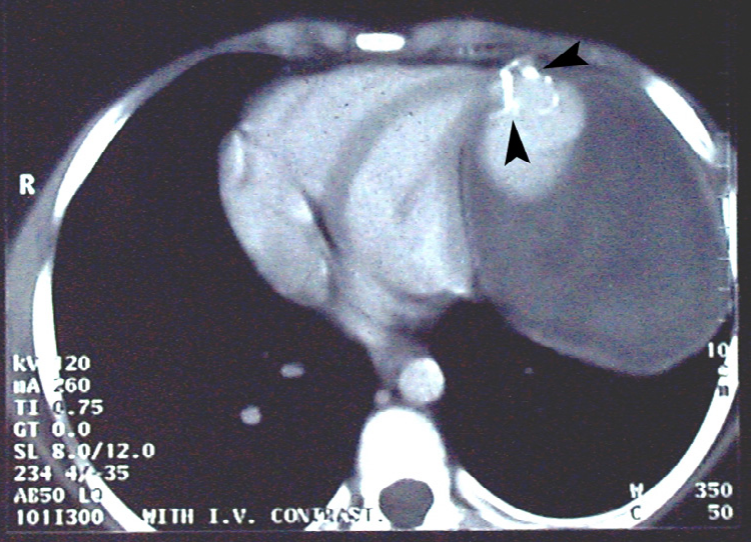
Chest computed tomography scan with contrast at the level of T7 showing the large pseudoaneurysm. The lesion was a well-defined partially calcified mass with tubular density adjacent to the heart. Arrowheads indicate the calcifications. It was filled with contrast medium concurrently with the heart. This lesion, which was mostly occupied by thrombosis, had a mass effect on heart.
